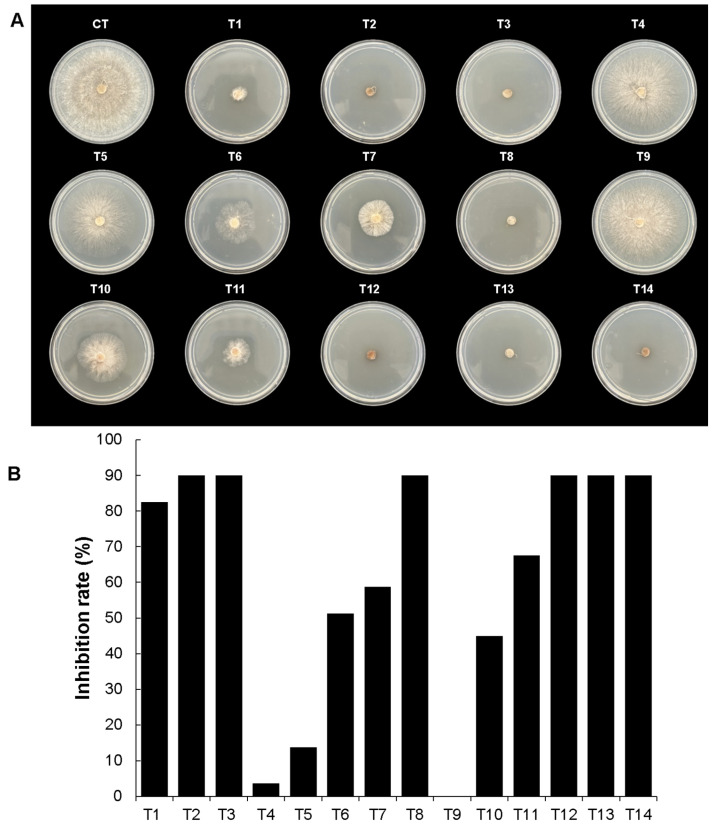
The mycelial growth inhibition rates of standard VOCs against *R. solani*. The treatments applied were T1, T2, and T3 with 2,5 dimethyl pyrazine concentrations of 100 µL, 150 µL, and 200 µL; T4, T5, and T6 with 100 µL, 150 µL, and 200 µL of acetoin; T7 and T8 with 10 µL and 20 µL of benzaldehyde; and T9, T10, and T11 with 50 µL, 100 µL, and 150 µL of 2-butanone. Also, the combination of pyrazine, acetoin, and benzaldehyde was used with different concentrations. T12 used 10 µL of each compound, T13 used 15 µL of each compound, and T14 used 10 µL of 2,5 dimethyl pyrazine and 20 µL of the other compounds. (A) The radial mycelial growth inhibition effects of the VOCs used against *R. solani*. (B). Percentages of mycelial inhibition with the mean value of two essays. CT mean is the control without treatment.
